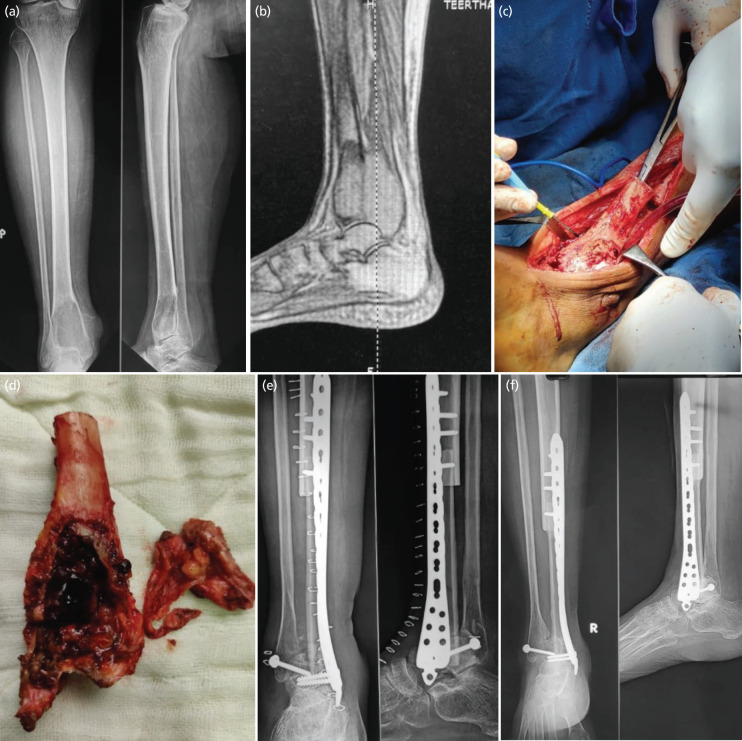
(a) Radiograph of the patient on presentation showing an expansile lytic lesion in the distal tibia involving the articular surface. (b) Magnetic Resonance Imaging confirming GCT and its soft tissue extension. (c) Intra-operative image of en bloc wide resection of the distal tibia tumour. (d) Resected distal tibia with tumour tissue. (e) Post-operative radiograph showing arthrodesis of the ankle using autologous fibular strut graft and fixation using a medial distal tibial Locking Compression Plate. (f) Two year postoperative radiograph showing radiological union at the proximal and distal graft sites.
